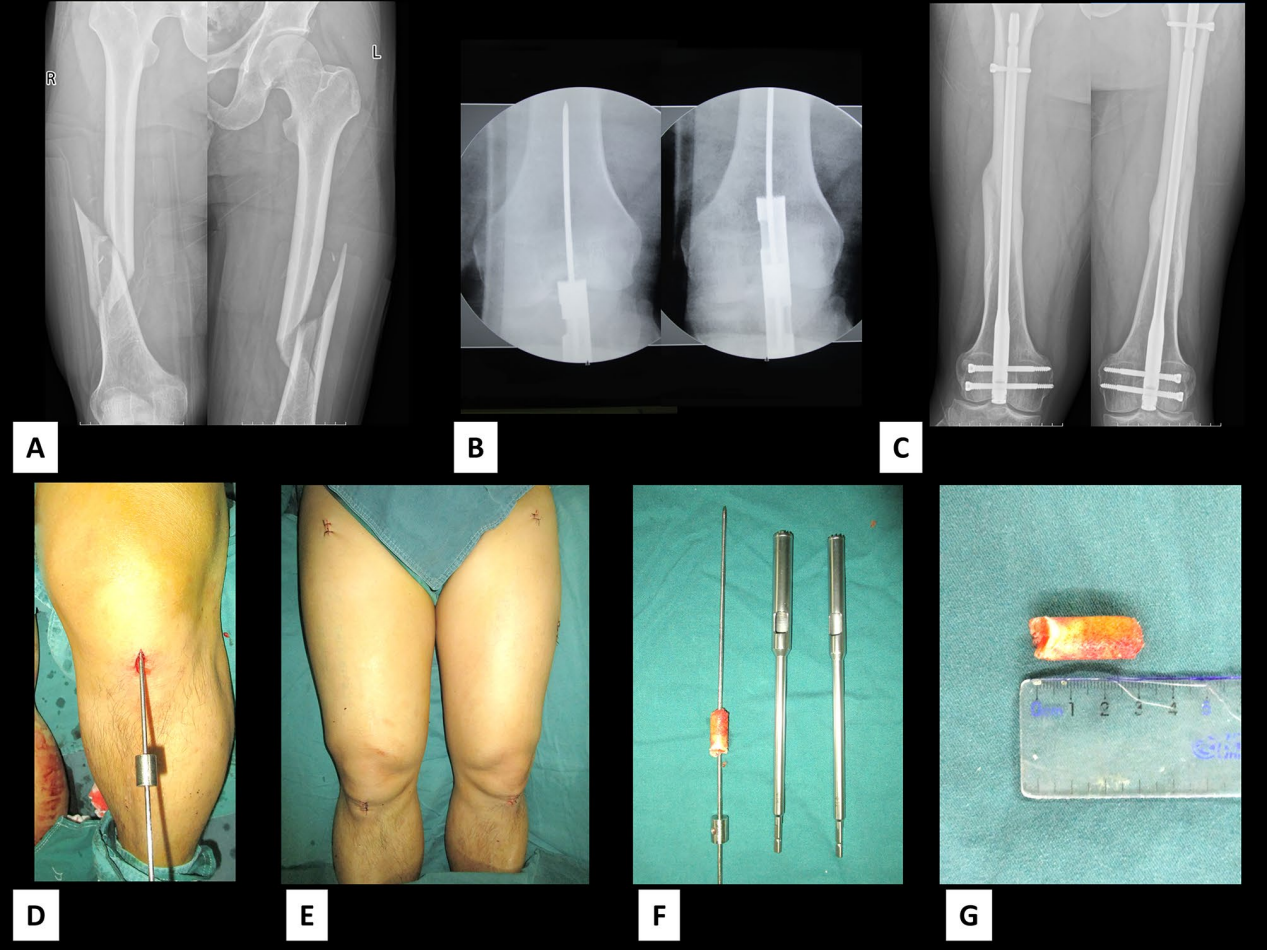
Application of a hollow trephine in a patient with bilateral femoral fractures A-C: Preoperative and postoperative anteroposterior radiographs of a patient D-G: Bone bolt obtained during surgery
